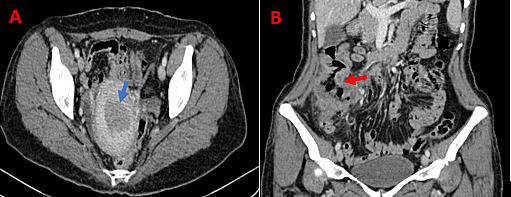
Abdominopelvic CT scan after contrast injection in axial section (A) showing thickening of the endometrium (blue arrow) and in coronal section (B) showing parietal thickening of the right colon (red arrow).
